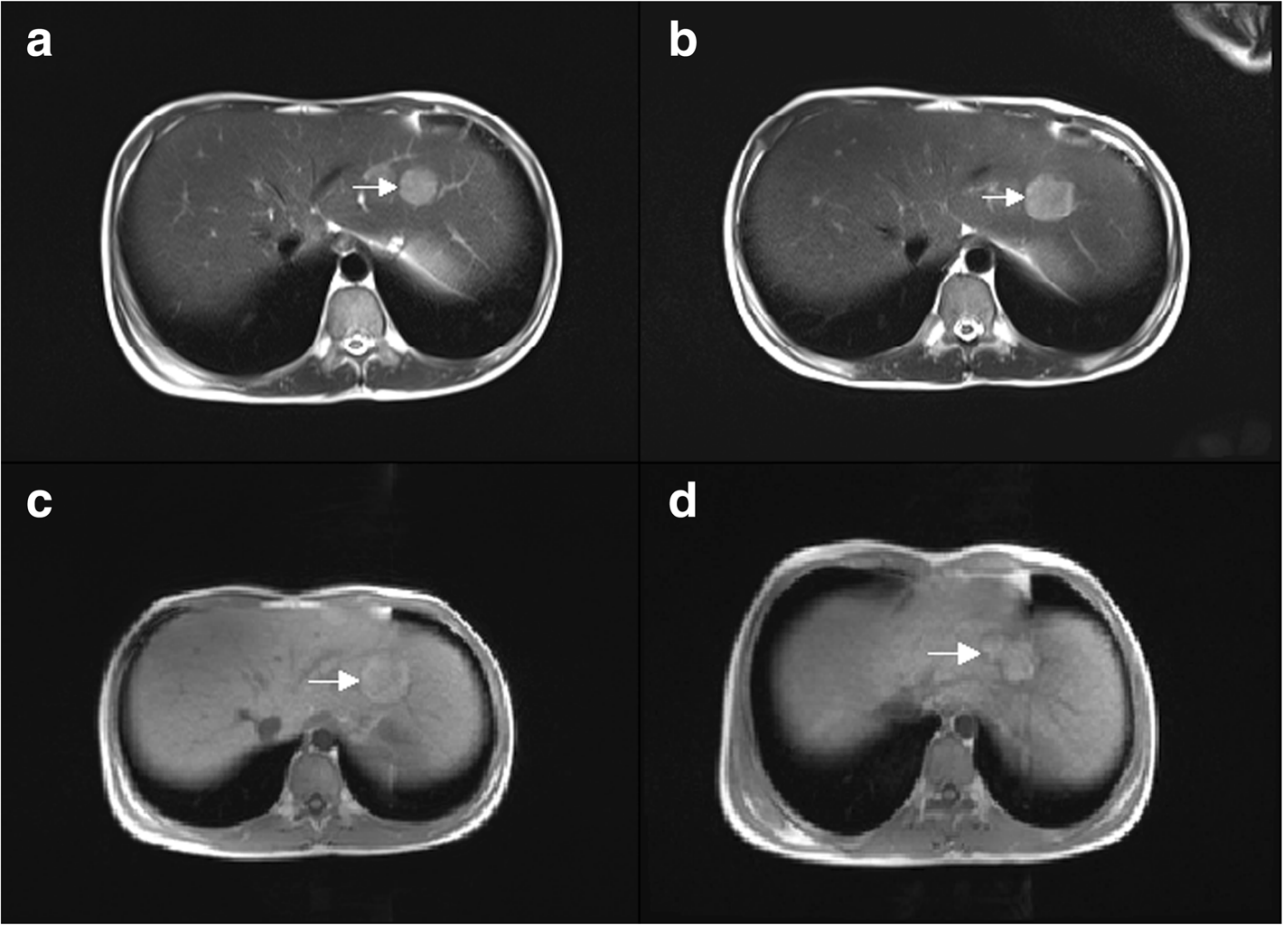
The Gd-EOB-DTPA tumor specific detection showed: glycogen accumulation. Arrow shows the largest nodule. a MRI (Gd-EOB-DTPA) examined on base line (2016.11.11 at presentation), the max size of nodules was 23 mm. b MRI (Gd-EOB-DTPA) examined 9 months after diagnosis of GSD I, the max size of nodules was 27 mm (c). MRI (Gd-EOB-DTPA) examined 12 months after diagnosis of GSD I, the max size of nodules was 34 mm. d MRI (Gd-EOB-DTPA) examined after radiofrequency ablation
